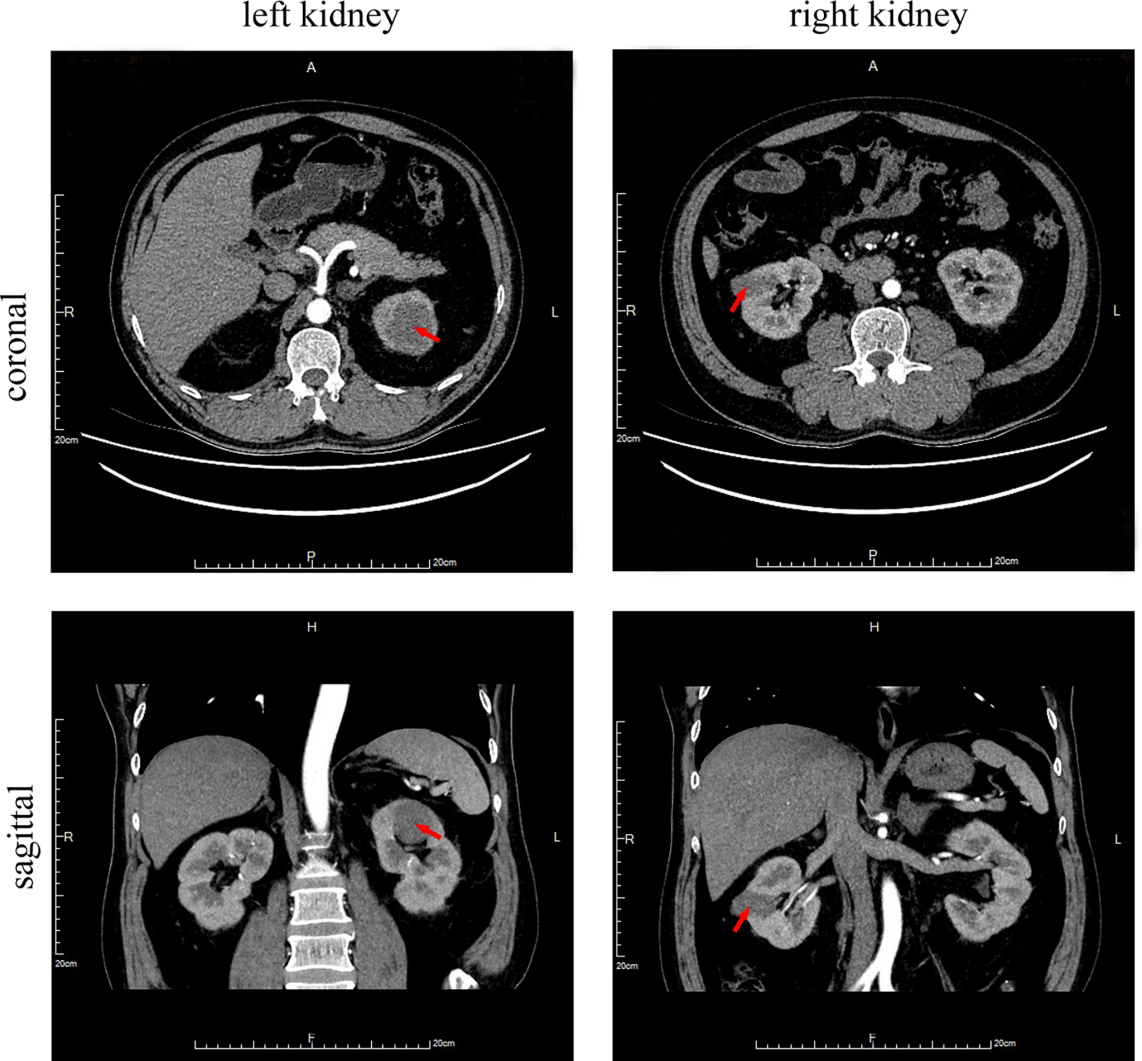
Abdomen CT scan with contrast media. Circular iso-density lesions of about 4.4 * 4.2 cm in the upper left kidney (arrow) and an oblong iso-density foci of about 3.4 * 2.1 cm in the middle of the right kidney (arrow).
